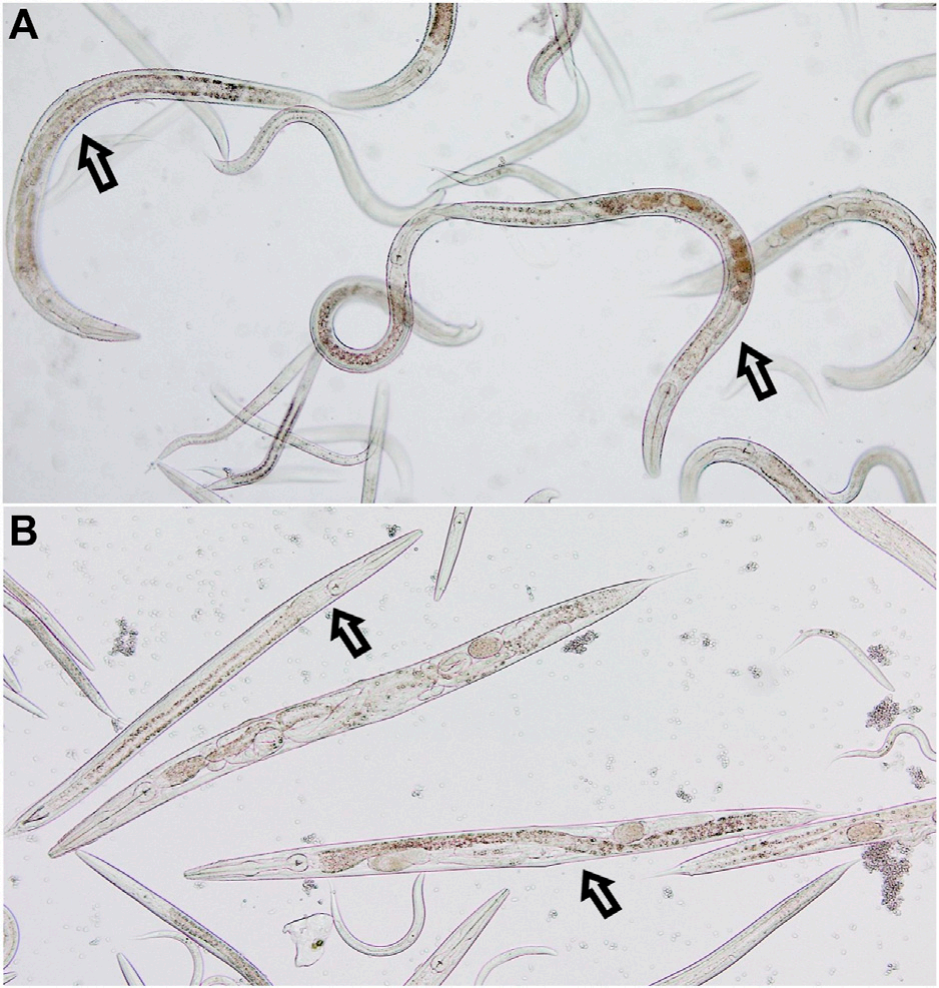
The culture of nematodes of the genus *Rhabditis*. (A) Control culture in 0.6% NaCl; (B) Culture after 24 h of exposure to the *Stachys sylvatica* L. extract obtained by ultrasonic-assisted extraction.
